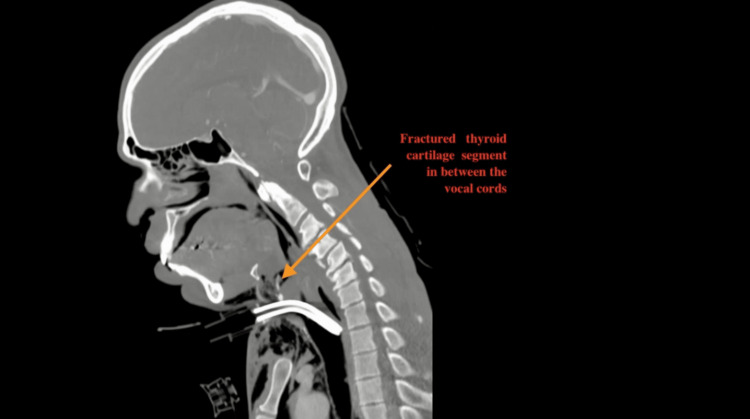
Pre-operative neck CT scan (sagittal view) The arrow shows the fractured thyroid cartilage segment separated from the thyroid cartilage.
